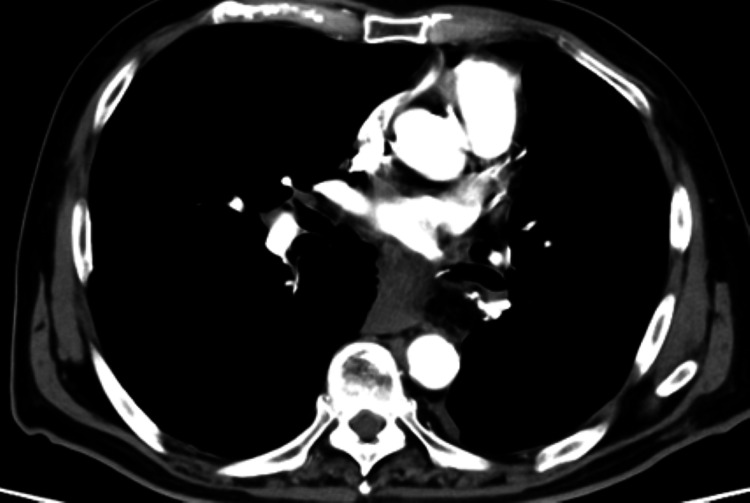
Follow-up contrast-enhanced computed tomography (CT). CT shows resolution of the thrombus in the left inferior pulmonary vein stump.
